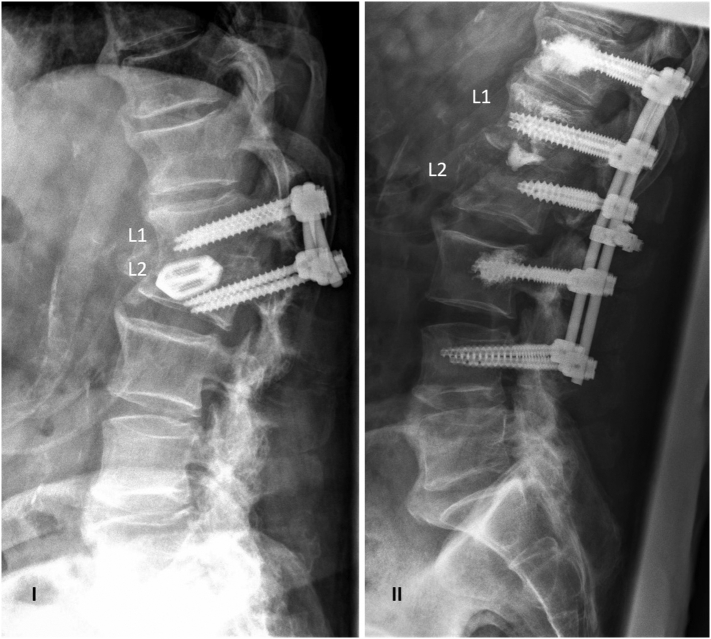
I. The upright lateral thoracolumbar radiography shows the construct failure with displacement of the pedicle screws and intervertebral cage, resulting in a loss of spinal alignment with segmental kyphosis L1/L2. II. Upright lateral thoracolumbar radiography after surgical revision with extended dorsal cement-augmented instrumentation and restoration of spinal alignment.
